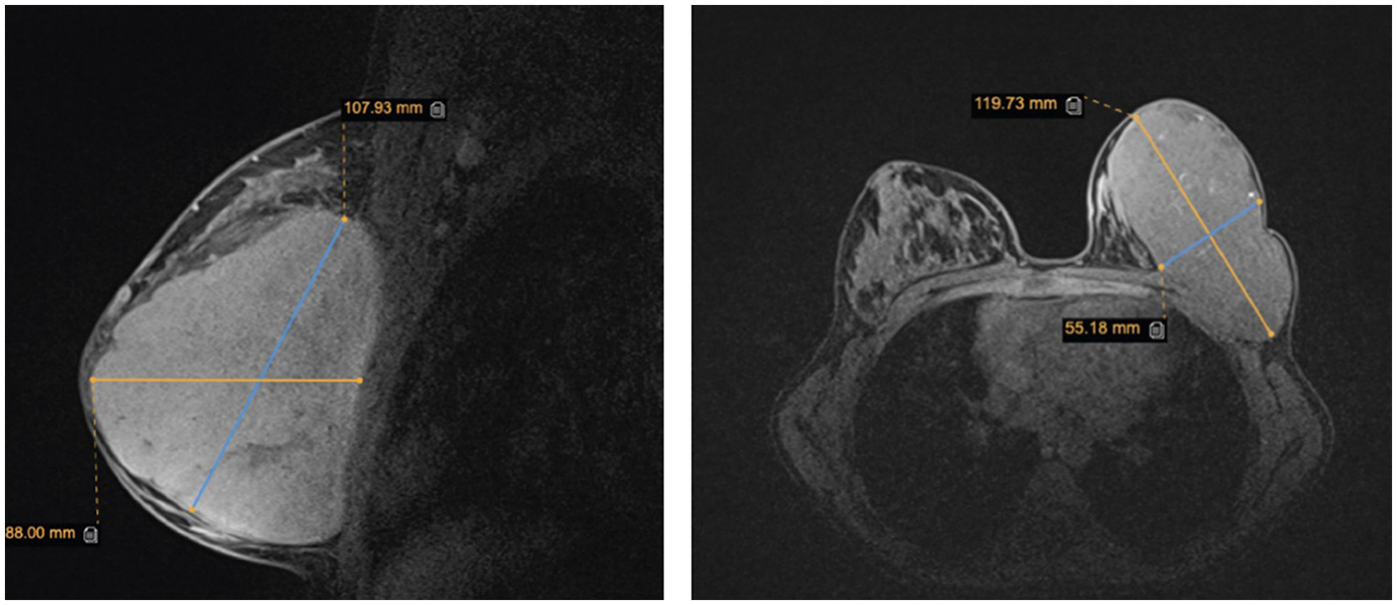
Breast magnetic resonance imaging. The image shows sagittal and axial views of a mass in the left breast.
